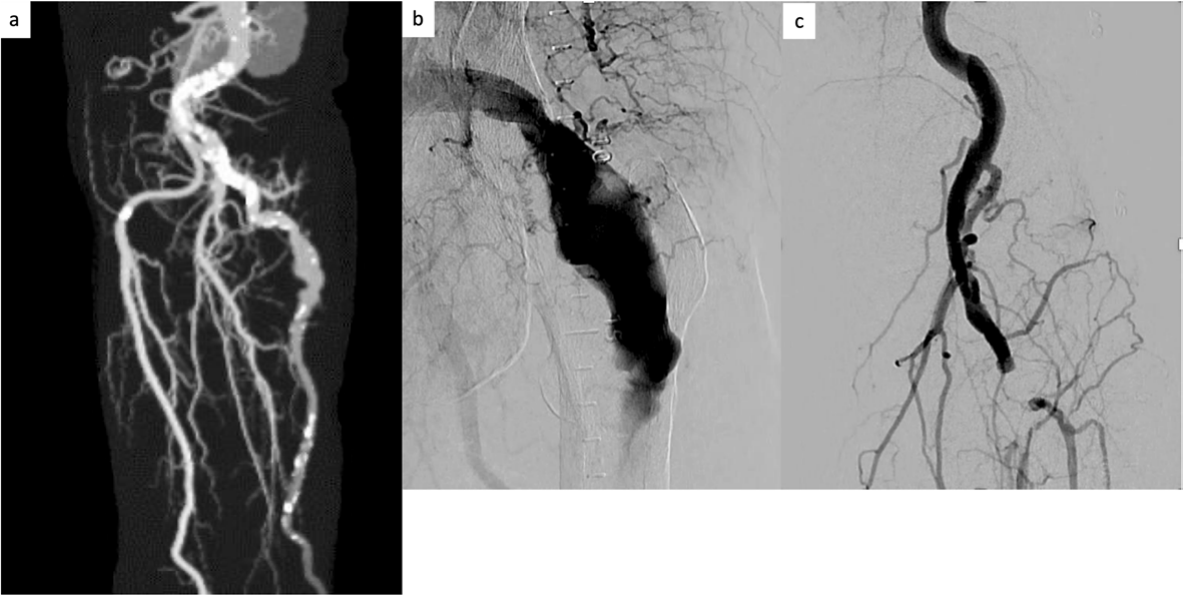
a Enhanced computed tomography angiography revealing a left PSA aneurysm and enhanced collateral flow toward the occluded PA below the knee joint, distal to a hypoplastic, incomplete SFA. b, c Angiography revealing aneurysmal dilatation of the proximal left PSA and total occlusion of the residual thrombus from the left distal PA to the tibioperoneal trunk. PSA, persistent sciatic artery; PA, popliteal artery; SFA
